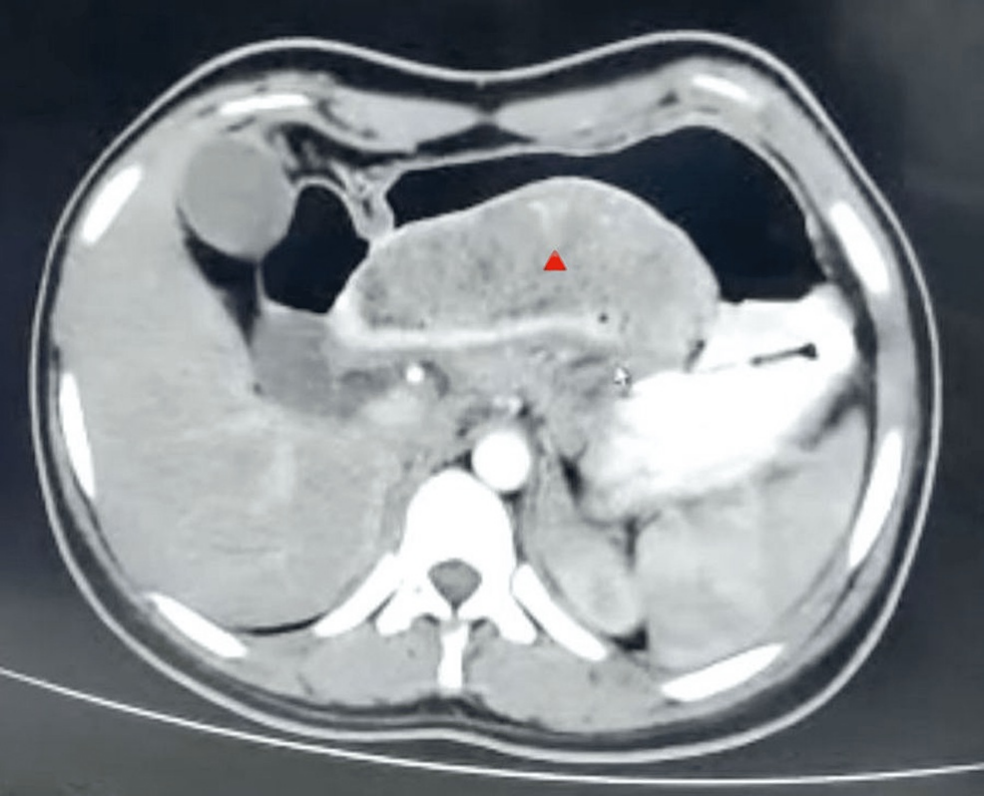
Trichobezoar in Duodenum, CT image Contrast-enhanced CT showed bezoar in the duodenum and dilated intestinal loops
